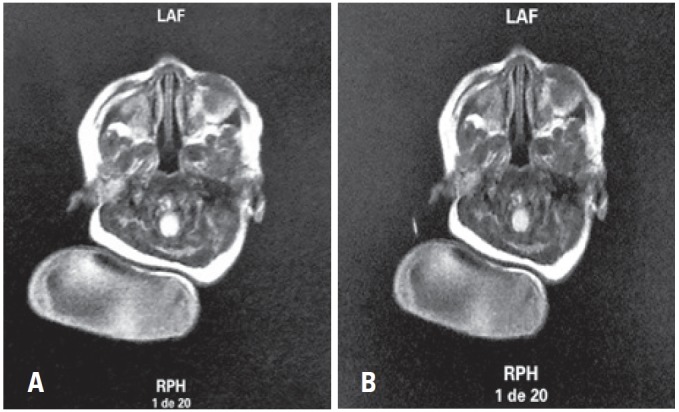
Cranial magnetic resonance imaging showing median suboccipital bone defect with extrusion of superior aspect and cerebellar vermis, cerebrospinal fluid and meninges (encephalomeningocele)
